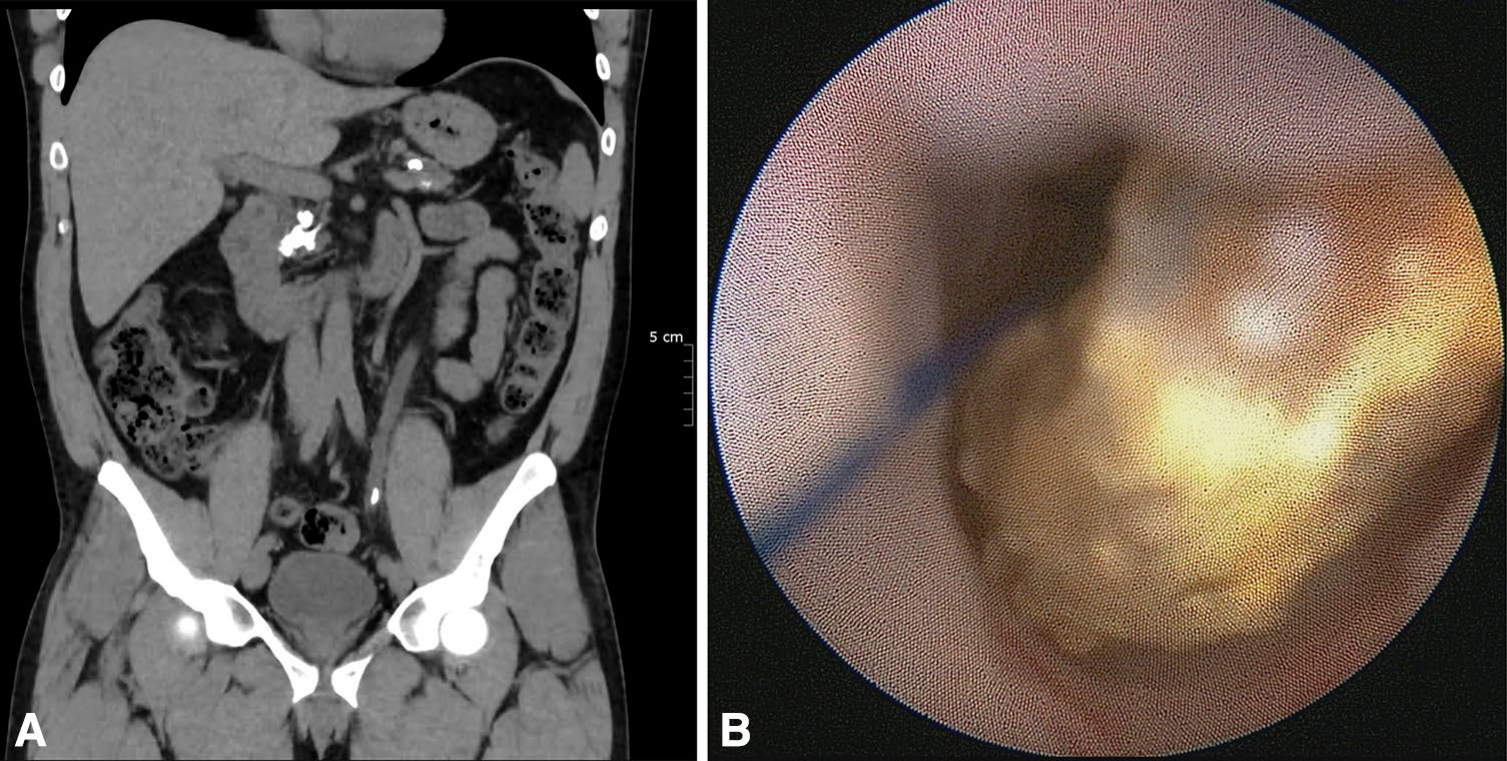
Patient with left ureteral stone and PSS. a Appearance of the stone along the suture in the dilated ureter on CT scan. b No ureteral inflammation is visible in contact with the stone (endoscopic appearance)
